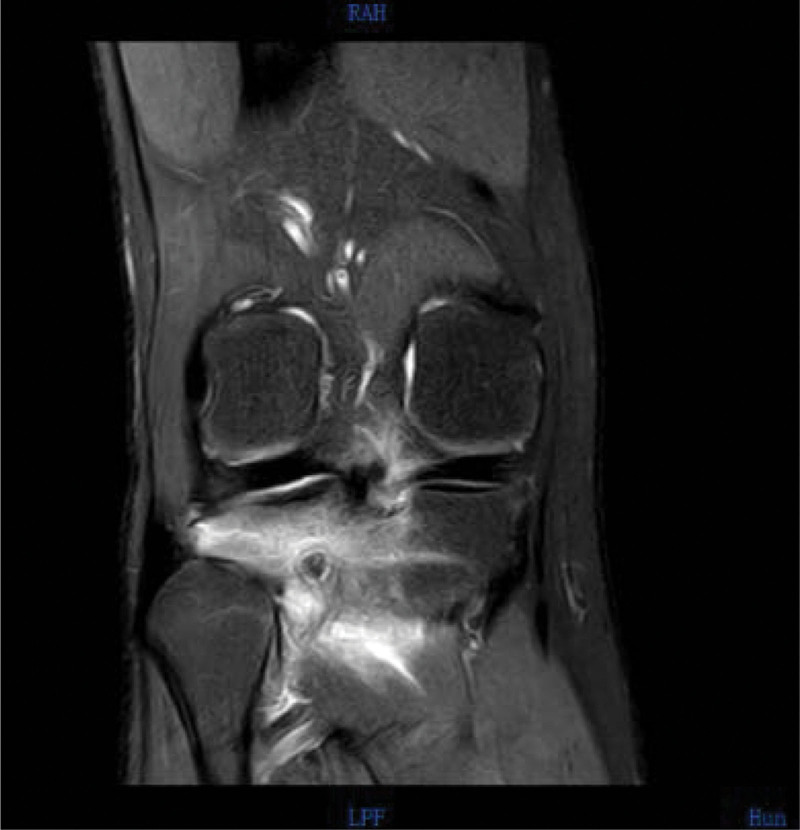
Preoperative MRI in coronal plane. MRI = magnetic resonance imaging.
